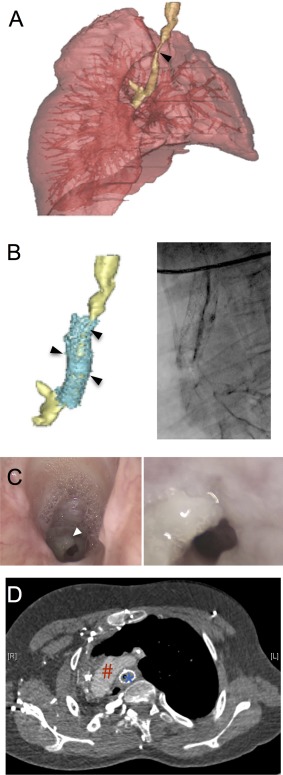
Preoperative evaluation of the airway demonstrating long‐segment tracheal stenosis with a single lung. (A): Three‐dimensional (3D) model of the tracheobronchial tree demonstrating extent of stenosis (arrow) and tortuous trachea with trifurcation of the airway. (B): 3D model (left) of the trachea demonstrating multiple stents (cyan) and their relation to the stenosis (arrows); corresponding bronchogram (right). (C): Endoscopic photograph of the airway at the level of subglottis (left) and at the stenosis (right). White arrow indicates granulation tissue. (D): Preoperative computed tomography angiogram demonstrating stenotic trachea with stent in relation to arch of aorta. Blue asterisk demonstrates stent location; red hashtag indicates arch of aorta.
